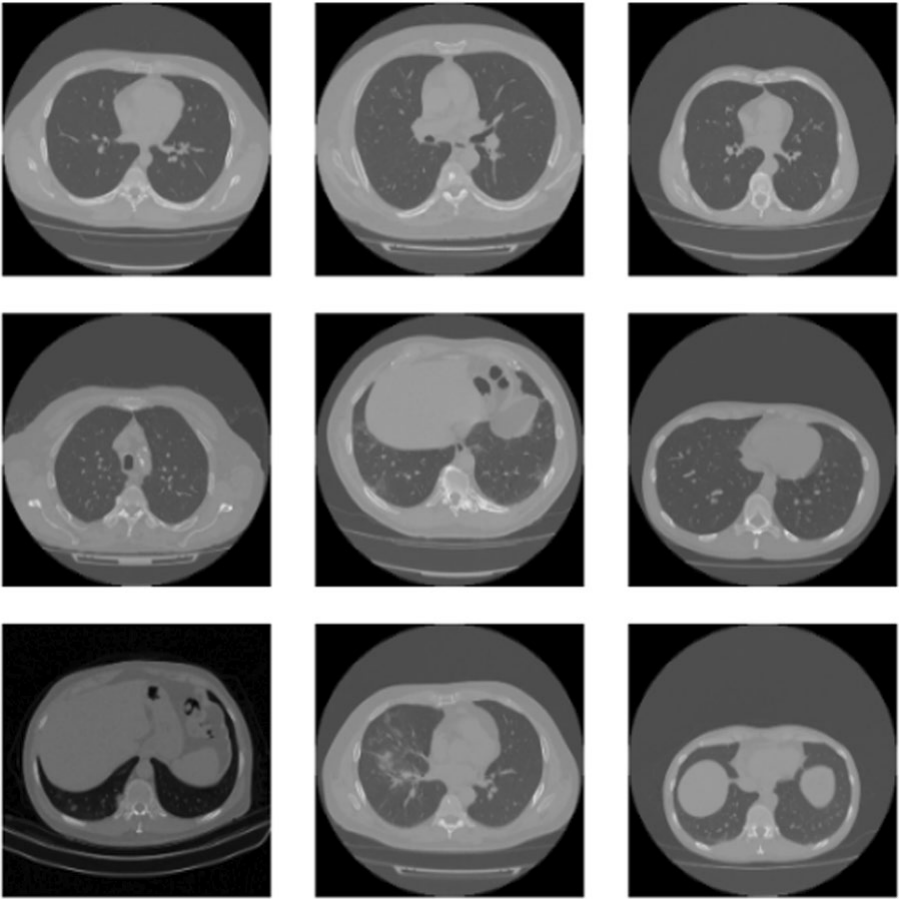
Samples from the Covid-19 CT scan dataset
